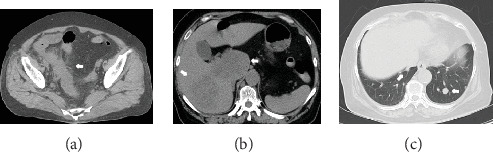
Pelvic CT showed inflammation spread and abscess formation around the cecum (a). Abdominal CT showed a huge mass and multiple nodules in the liver (b). Chest CT showed nodules in the lungs (c). Arrows: lesions.
